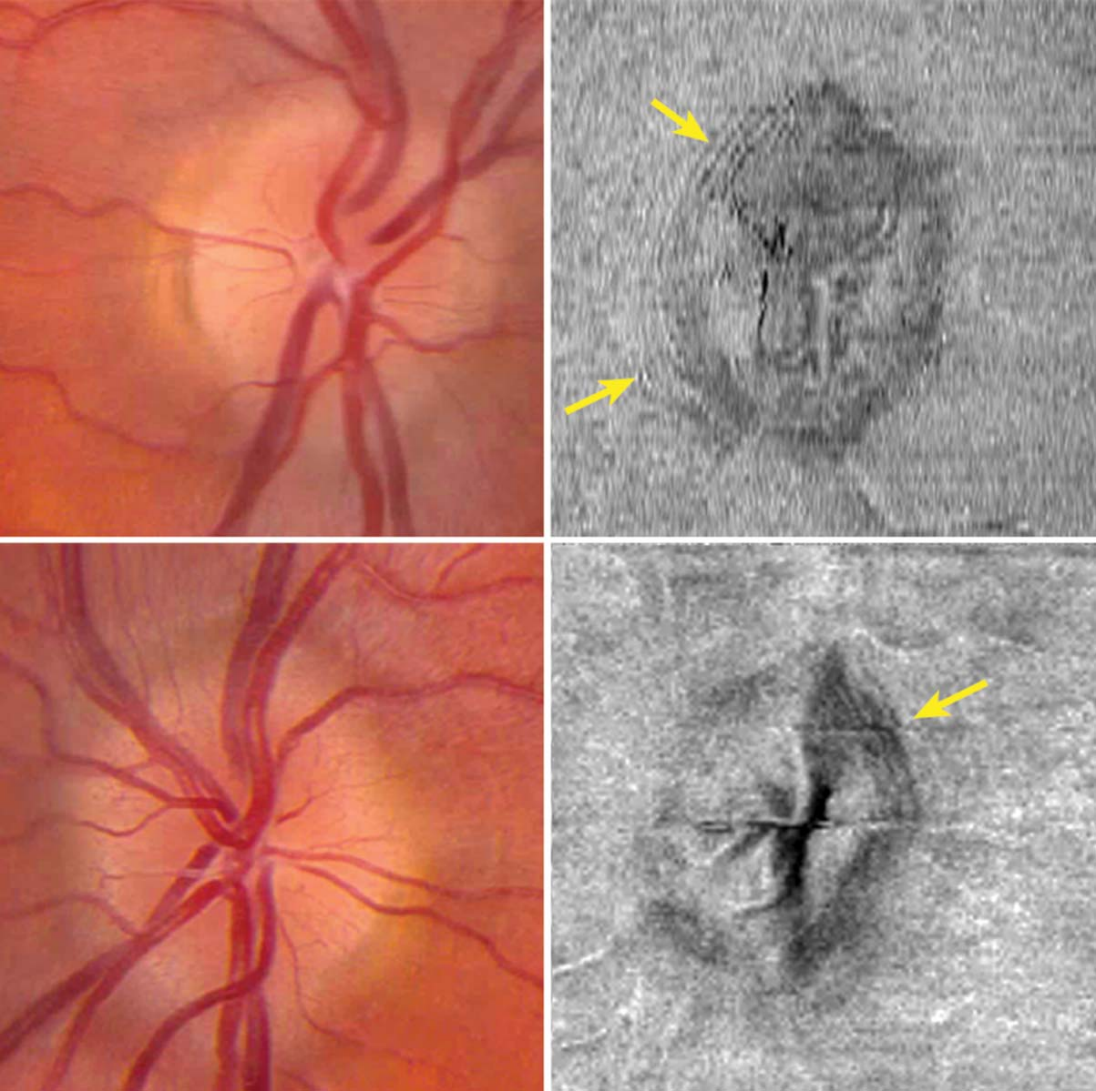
A 31-year-old woman with occasional headaches for 2 years, body mass index 30.5. Examination was otherwise normal. No drusen by B-scan or OCT; the mean RNFL thickness was 106 μm in the right eye, 101 μm in the left eye; no anterior deformation; en face OCT showed peripapillary wrinkles temporally in both eyes (*yellow arrows*). MRI/MRV was normal. Cerebrospinal fluid pressure was 33 cm. Some patients adapt to long-standing intracranial hypertension with minimal swelling, preservation of vision, in effect a chronic “compensated” papilledema. OCT, optical coherence tomography; RNFL, retinal nerve fiber layer. MRV, Magnetic resonance venography.
